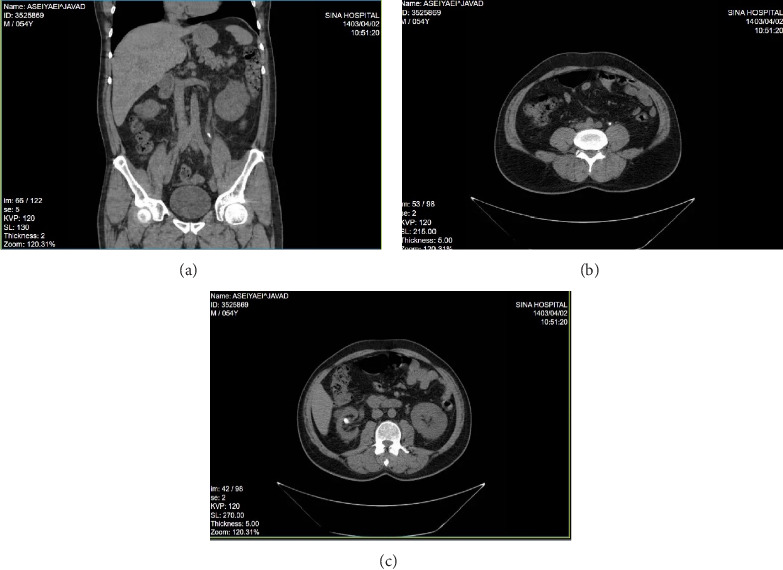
(a–c) Coronal and axial cut of noncontrast CT that revealed a 15 × 8 mm stone in the midureter of the left kidney, causing severe hydronephrosis (a-b). Axial cut of noncontrast CT that revealed a 13 × 10 mm stone in the left renal pelvis, causing mild hydronephrosis (c).
